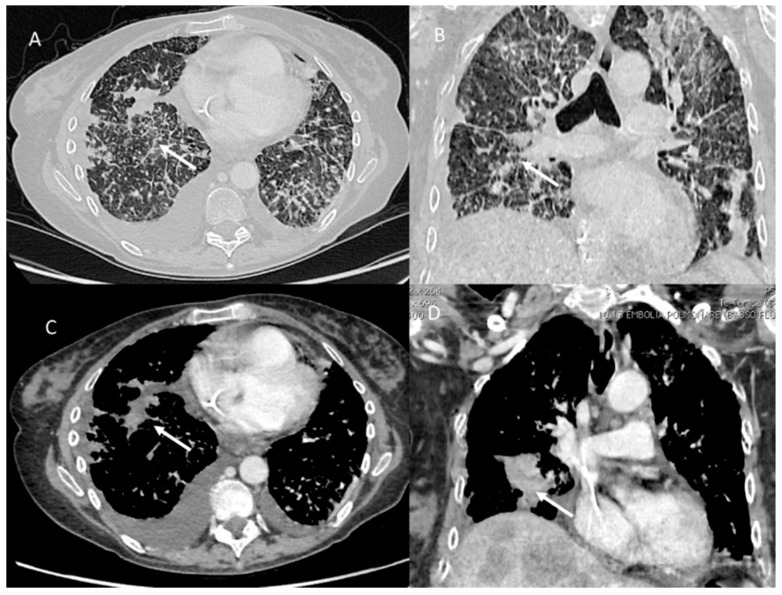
PLC in pancreatic cancer patient. CT (axial (A,C) and coronal (B,D)) shows comprise diffuse intrapulmonary infiltrates (arrows) with irregularly interlobular septal thickening, nodular thickening and pleural effusions.
